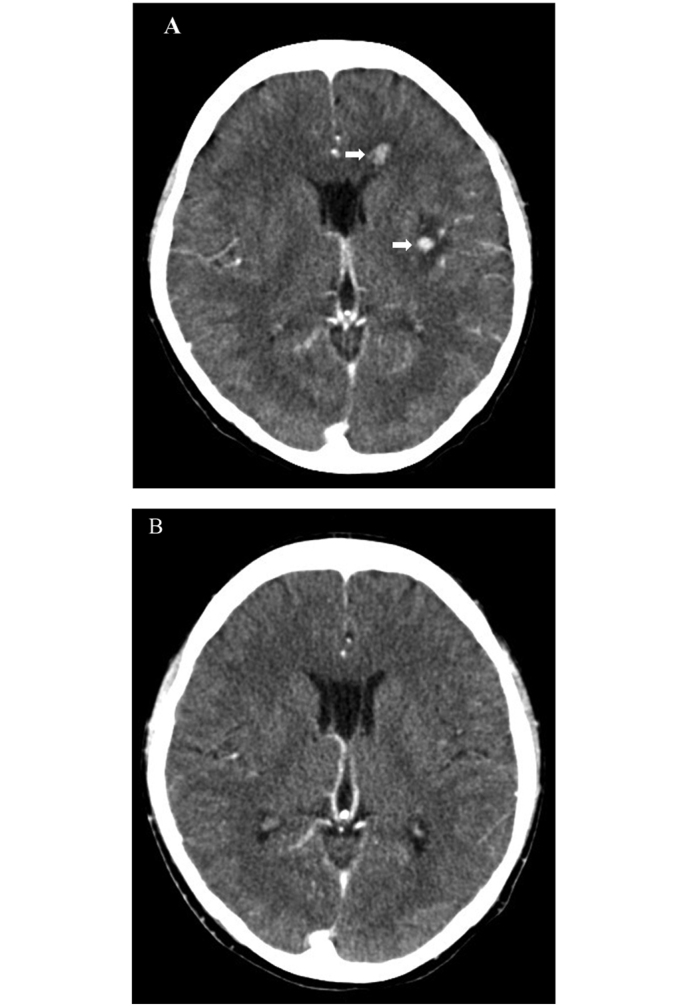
Computed tomography scan of the brain. (A) Multiple small enhancing nodules (white arrows), with mild perifocal edema in the bilateral cerebrum and left cerebellum. (B) Normal gray and white matter attenuation without evidence of abnormal enhancing lesions, demonstrated following dasatinib treatment and whole brain radiotherapy.
